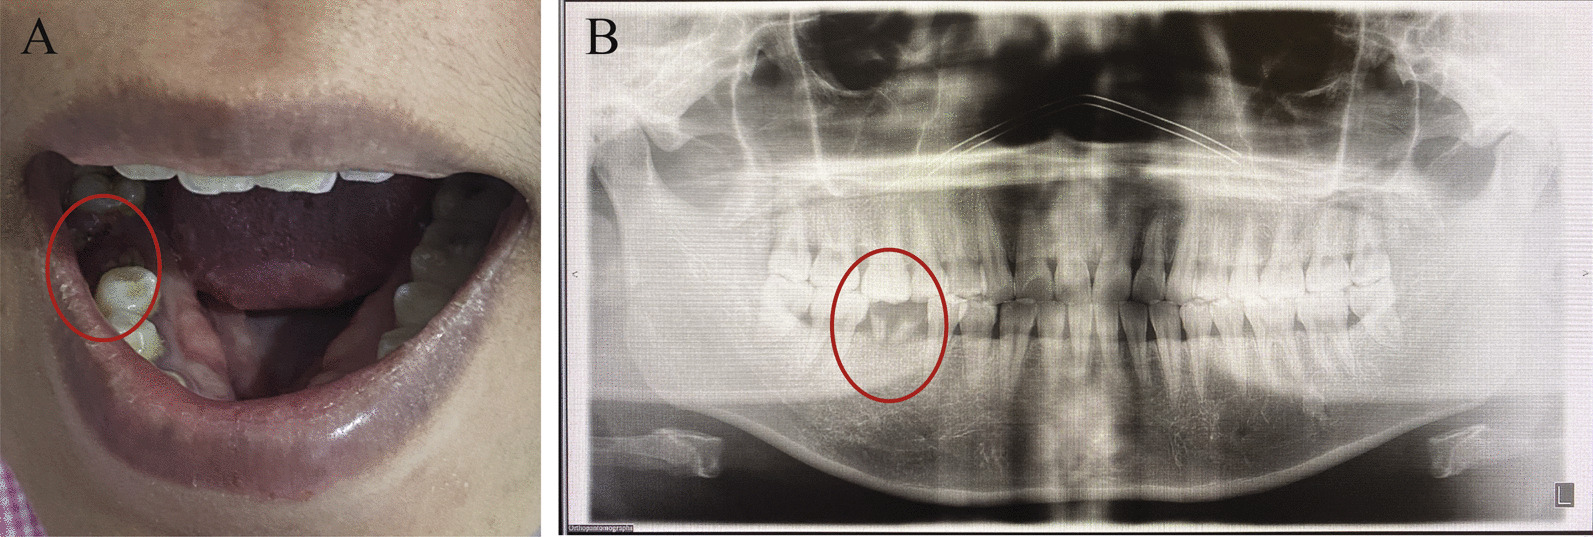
A Pulp necrosis with acute apical periodontitis, deep dental carries; B Dental X-ray: Fracture right lower molar tooth
